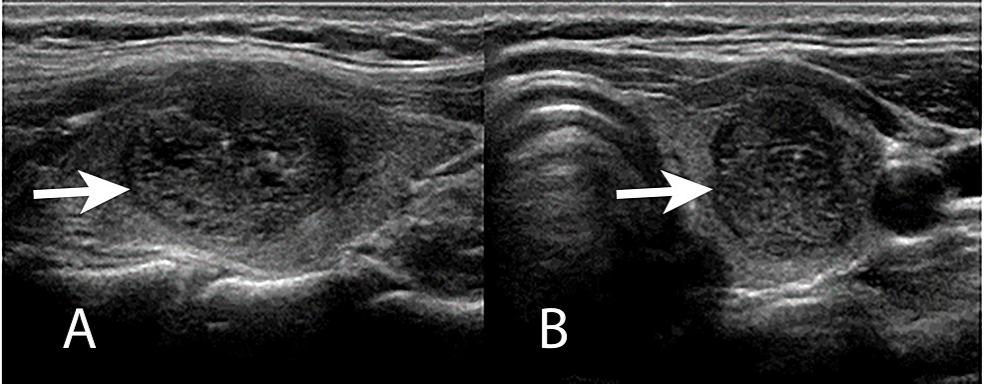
Left thyroid ultrasound (A) Sagittal view. (B) Transverse view. Bold arrows point to the nodule.
